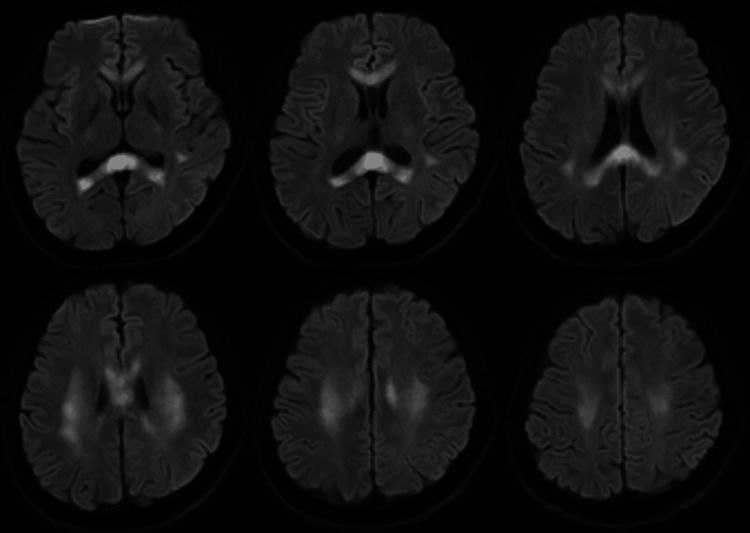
Initial brain MRI showing high signal intensity on DWI sequences in the splenium of the corpus callosum and bilateral frontal-parietal white matter. MRI: magnetic resonance imaging; DWI: diffusion-weighted imaging
